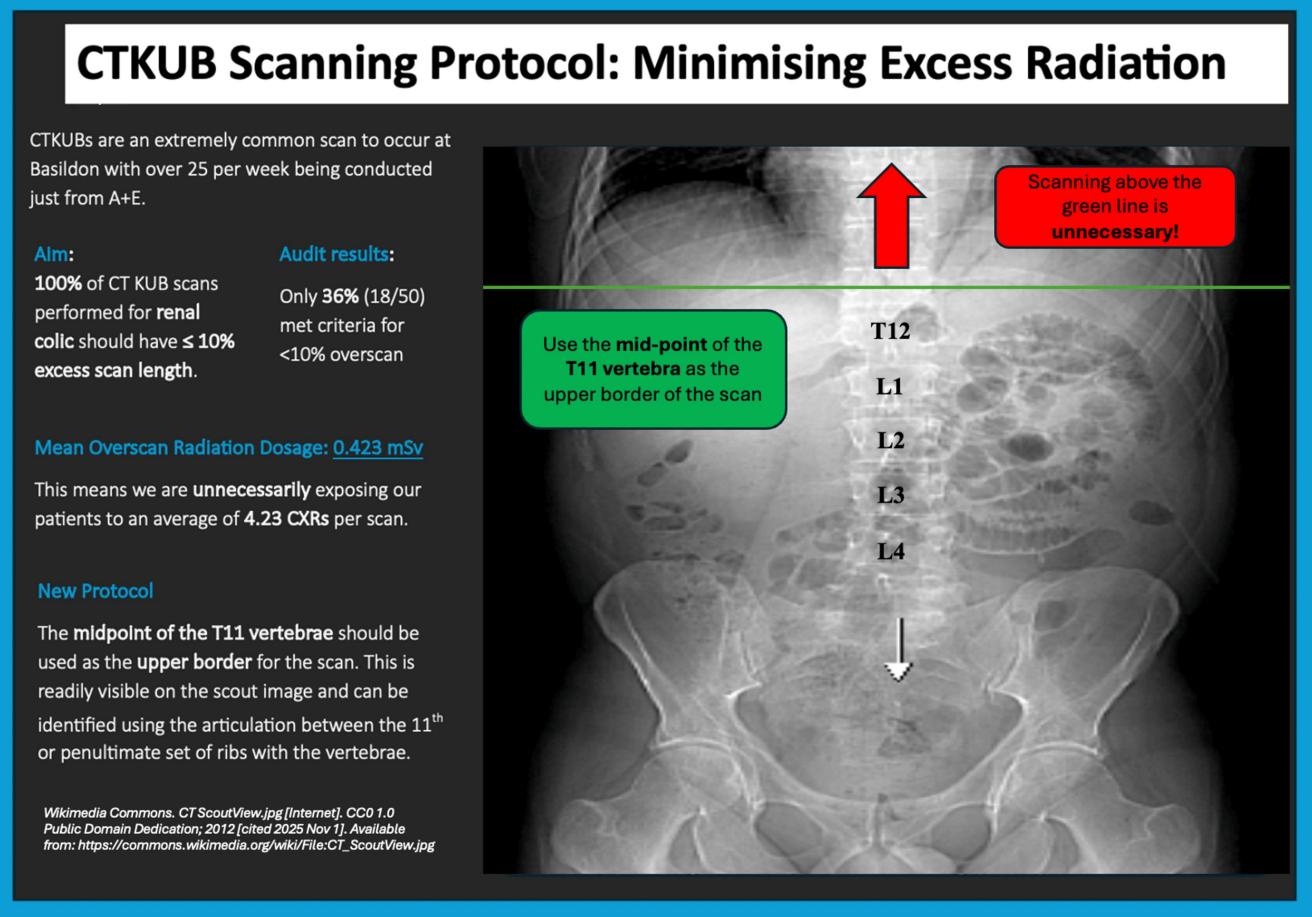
Poster demonstrating first-cycle audit findings and instructions for the new protocol, which was displayed in each CT control room within the hospital.
